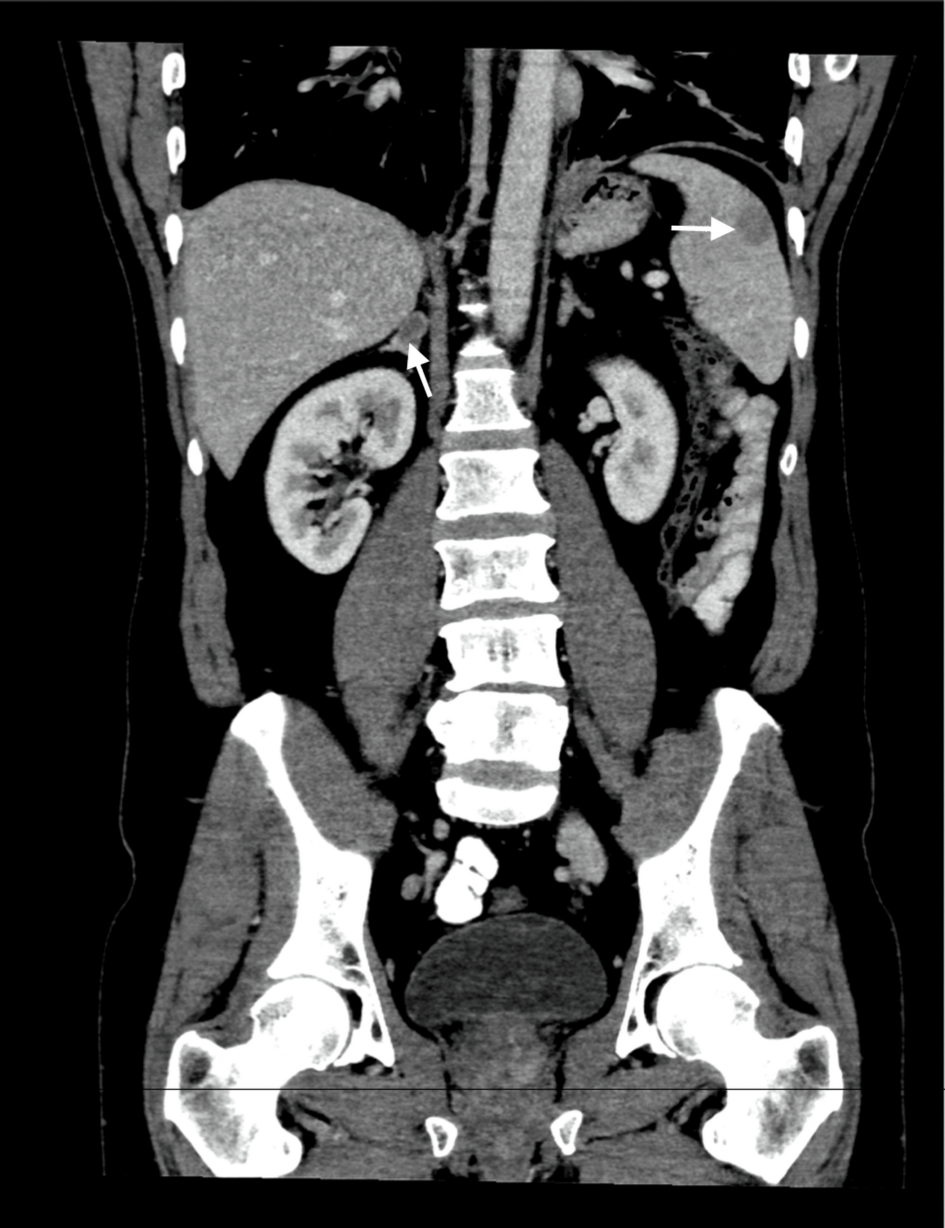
Contrast-enhanced CT scan of the abdomen and pelvis Solitary splenic and right adrenal nodules are visualized (arrows). These findings initially suggested sarcoidosis because the pattern of symmetrical lymphadenopathy and perilymphatic nodularity is typical of granulomatous disease. CT: computed tomography
